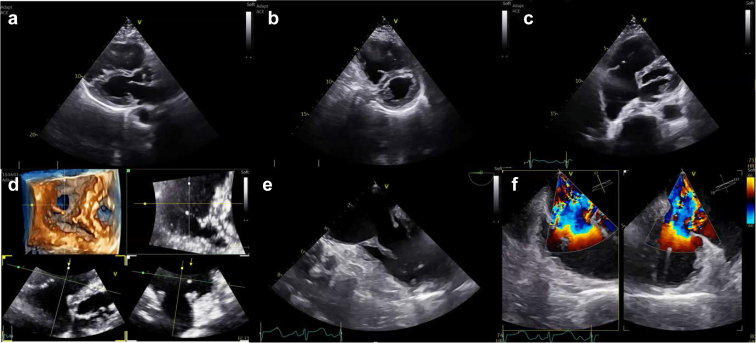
Computed tomography analysis for TTVR screening using the Lux-Valve Plus system – Part 1. (a) The annular area, perimeter, and maximum and minimum diameters are measured in diastole to determine the appropriate device size and percentage oversizing. (b) Dimensions of the fabric skirt plane are also measured. (c and d) The target positions of the device and septal anchor are modeled. (e) The distances between the center of the tricuspid annulus and the free wall of the right ventricle and the right ventricle are measured Abbreviation: TTVR, transcatheter tricuspid valve replacement.
